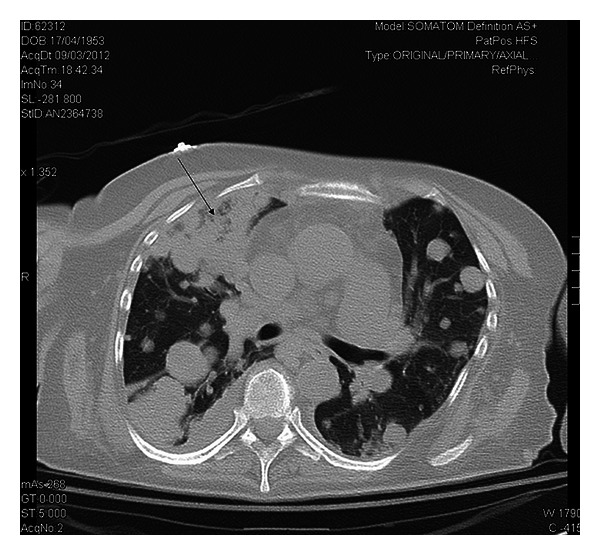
Lung abscess. Chest high-resolution computed tomography pulmonary angiography showing in the right upper lung lobe an area of consolidation with air inside a cavitary lesion (lung abscess probably) (arrow).
